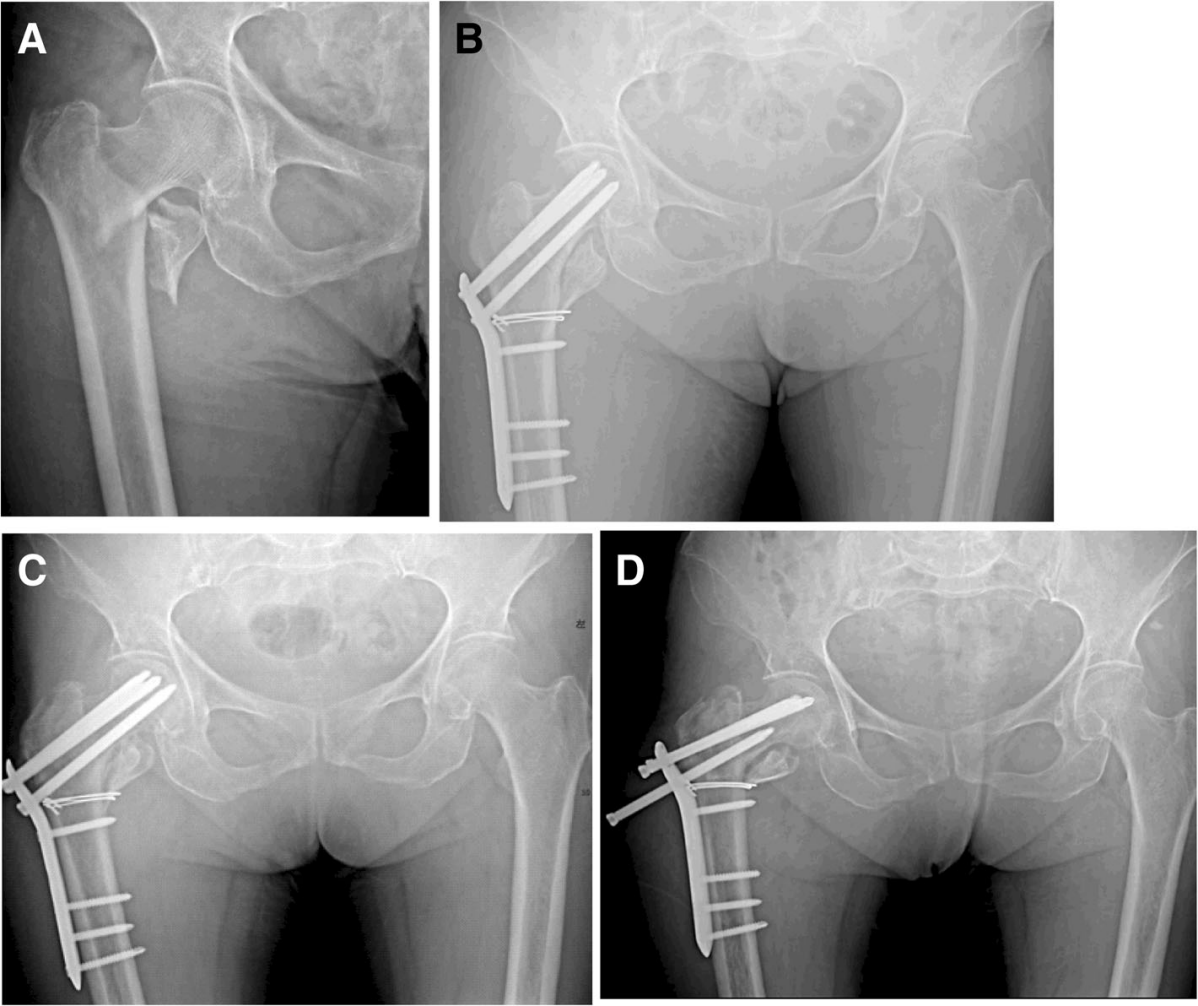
A 81-year-old female with AO/OTA type 31A2.1 experienced loosening of proximal screws. a Radiograph after injury. b Postoperative radiograph showing good reduction and fixation (1 week after surgery). c Ten weeks postoperative radiograph showing loosening of proximal femoral screws, loss of reduction and varus collapse. d Progressive loss of reduction, screws loosening and varus collapse (24 weeks after surgery)
